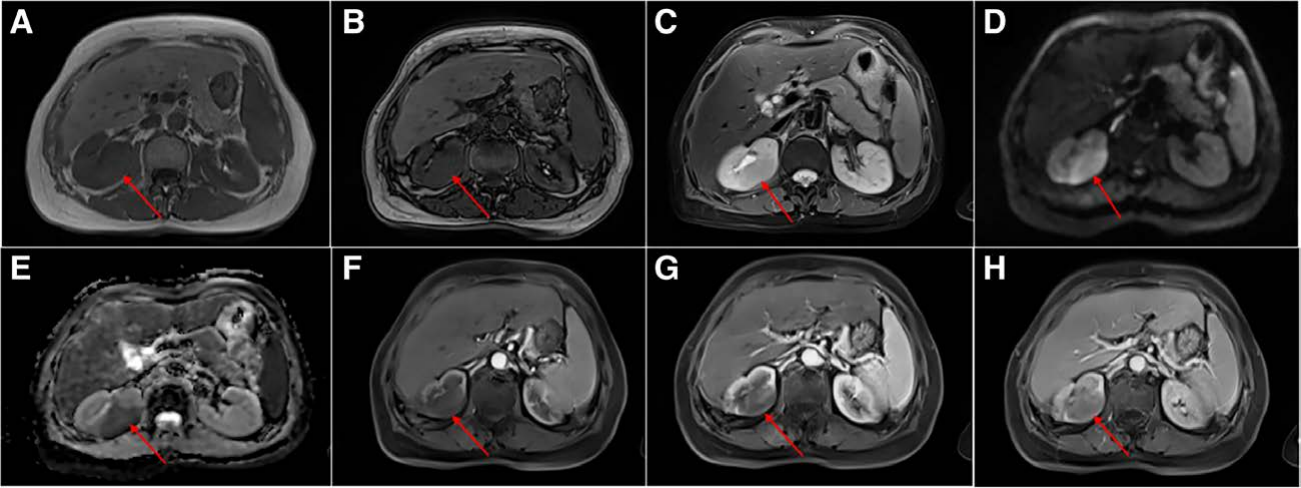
Magnetic resonance imaging (MRI) (slice thickness 6.5 mm). (A) In-phase (arrow). (B) Out-phase (arrow). (C) T2-wighted image shows low signal intensity (arrow). (D) DWI (arrow). (E) ADC (arrow). (F) Arterial phase (arrow). (G) Venous phase (arrow). (H) delay phase (arrow). ADC = apparent diffusion coefficient.
